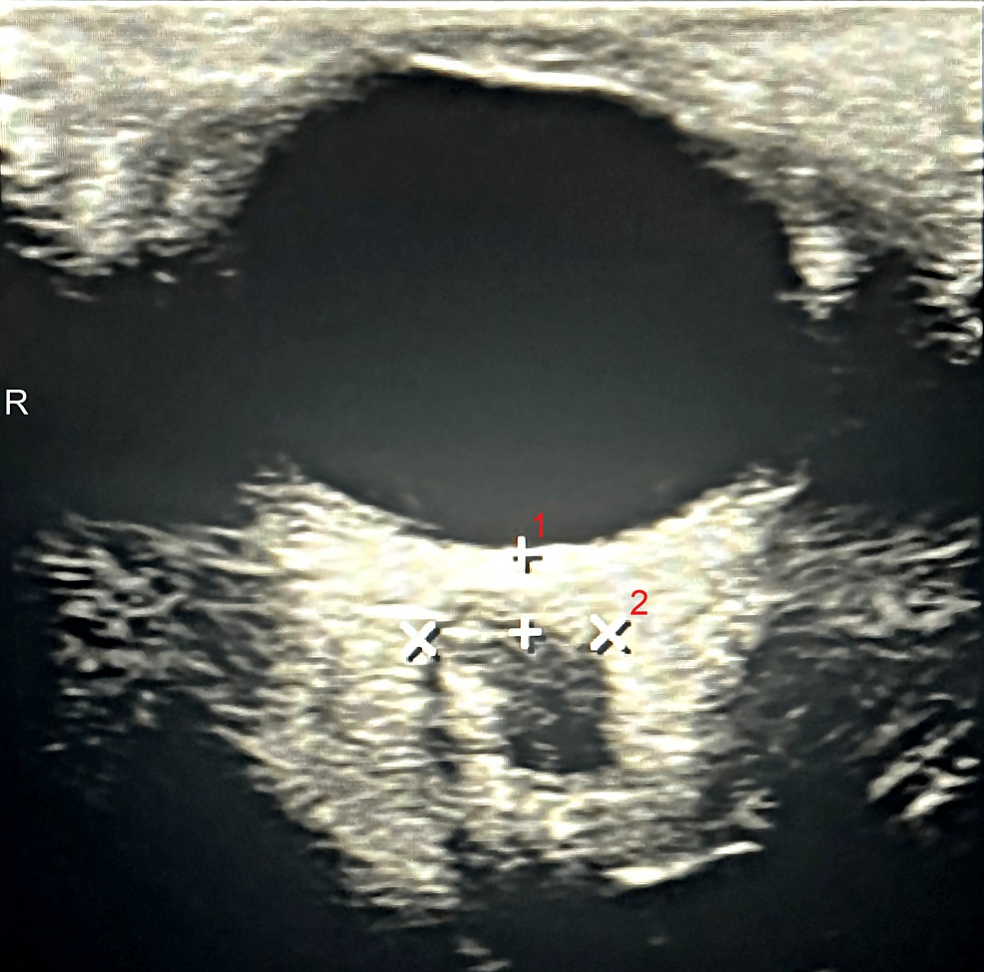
Optic Nerve Sheath Ultrasound The diameter of the optic nerve sheath measured 3 mm posterior to the globe of the eyeball (distance 1). The ultrasound image is obtained via a transverse view through the left eye. The transverse diameter of the optic nerve sheath was found to be 0.738 cm (distance 2).
